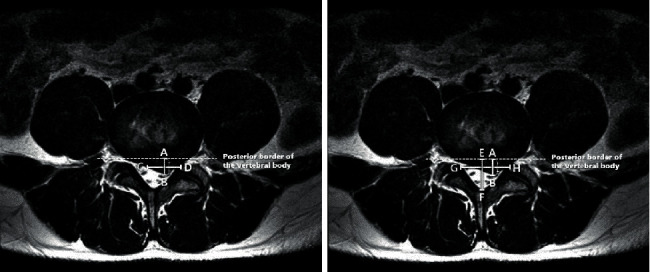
Methods for calculating the herniation index (HI) in the axial plane of the magnetic resonance imaging (MRI) scan. AB: the maximal anteroposterior disc length, which is the diameter of the herniated disc material extended maximally from the posterior border of the vertebral body. CD: the width of the herniated disc material at the level of the middle AB distance of the herniated disc material from the coronal plane of the MRI scan. EF: the maximal anteroposterior canal length. GH: mid-AB distance. HI was calculated using the following formula:
